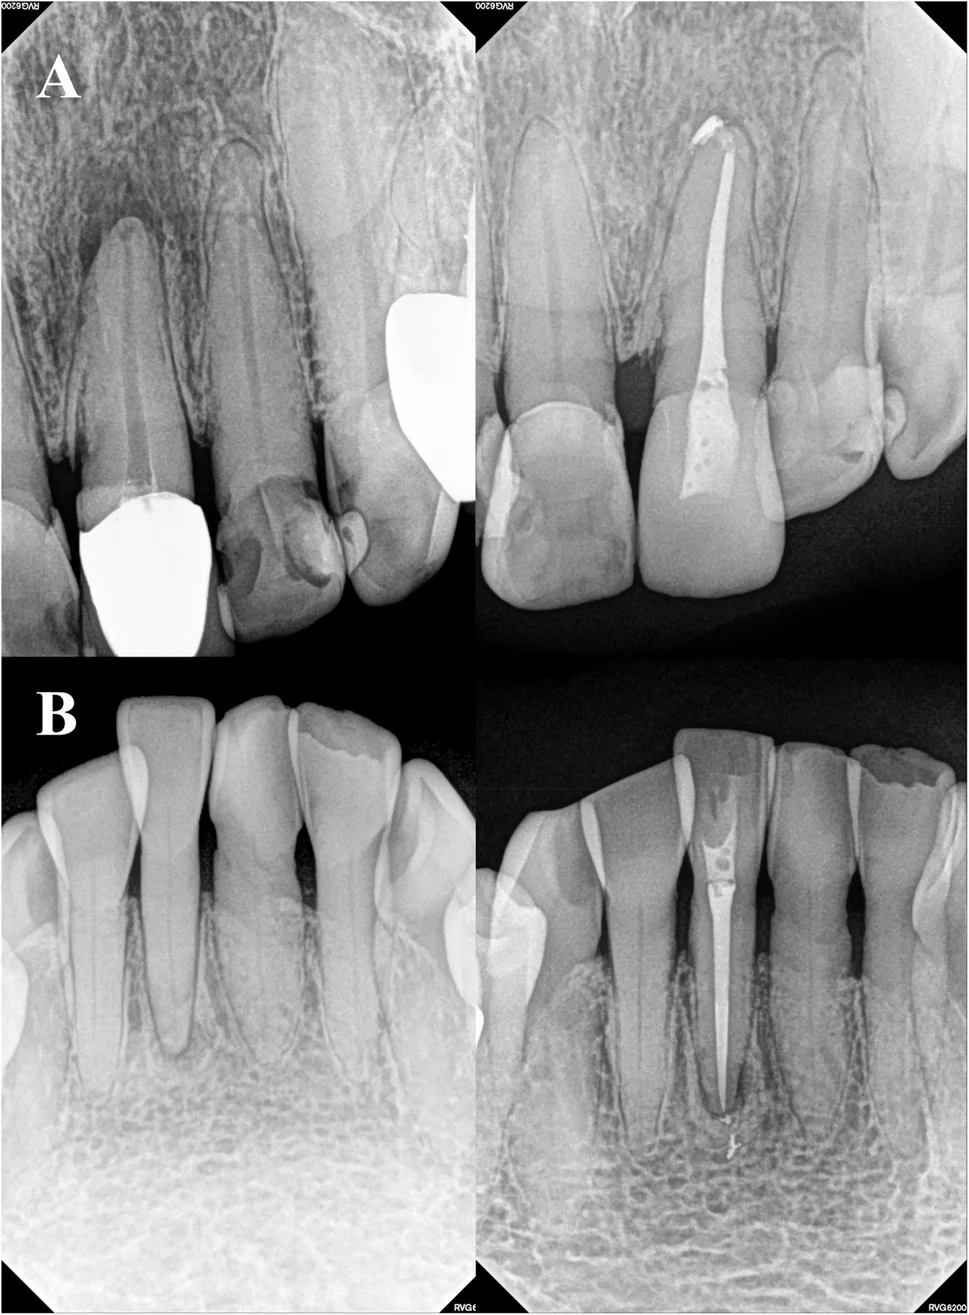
Representative healed and diseased cases demonstrated by periapical radiographs. A Healed case: initial pre-operative Periapical Index (PAI) score of 5 (left) decreased to a final PAI score of 2 (right) after treatment, indicating substantial resolution of the periapical lesion, bone regeneration, and a favorable overall treatment outcome. B Diseased case: initial pre-operative PAI score of 3 (left) remained unchanged at a final PAI score of 3 (right) after treatment, demonstrating persistent periapical radiolucency at follow-up and an unfavorable overall treatment outcome
